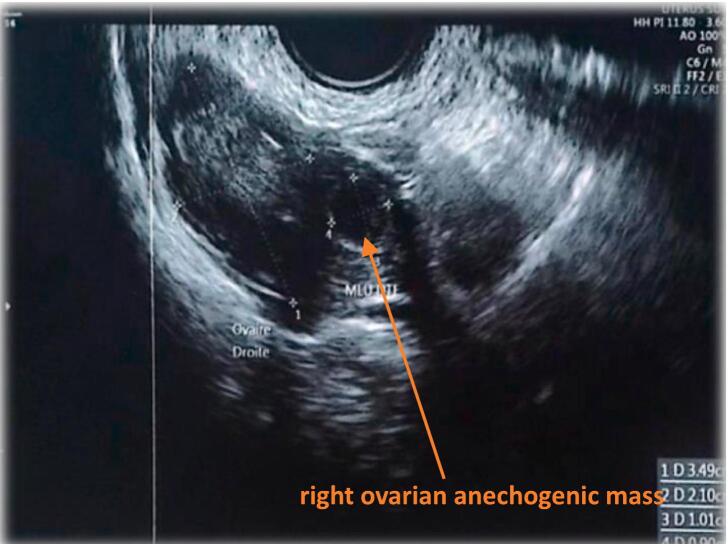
Transvaginal ultrasound showing the right ovarian mass.
